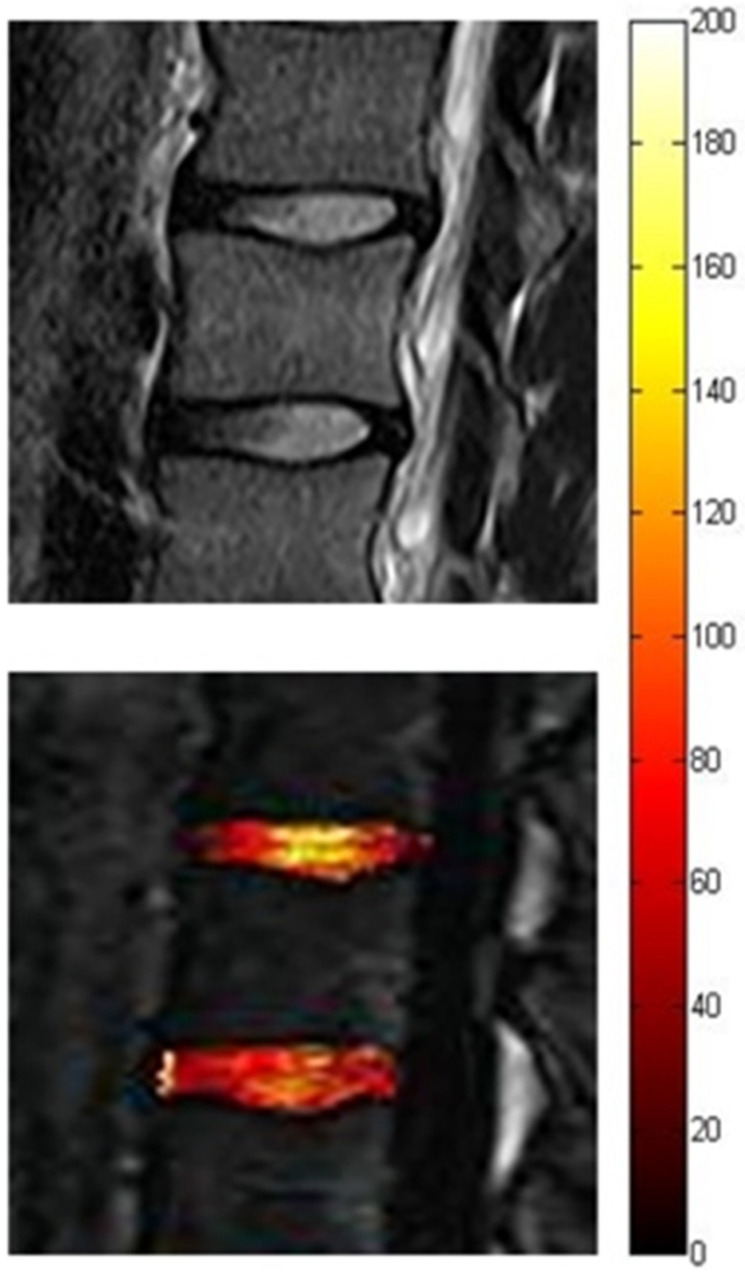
Color-coded map based on T1ρ images (lower panel) showing early disc degeneration (in red) at the levels L3–L4 relative to L2–L3, despite a similar signal intensity of the two discs in the T2-weighted image (upper panel).
